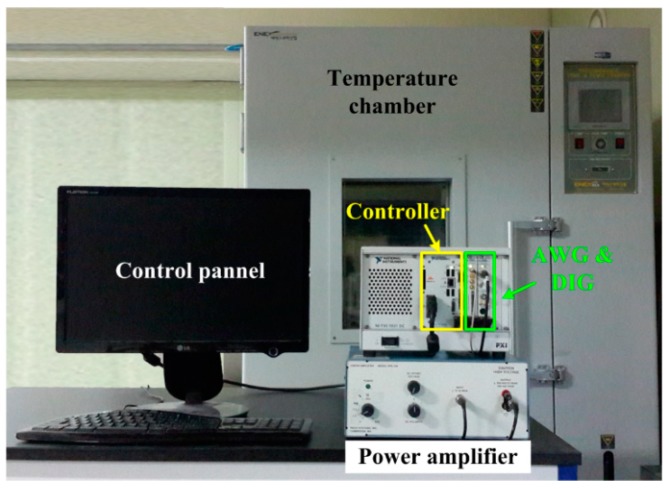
Self-sensing nonlinear ultrasonic system for temperature variation tests. AWG: arbitrary waveform generator; DIG: 14-bit digitizer.
